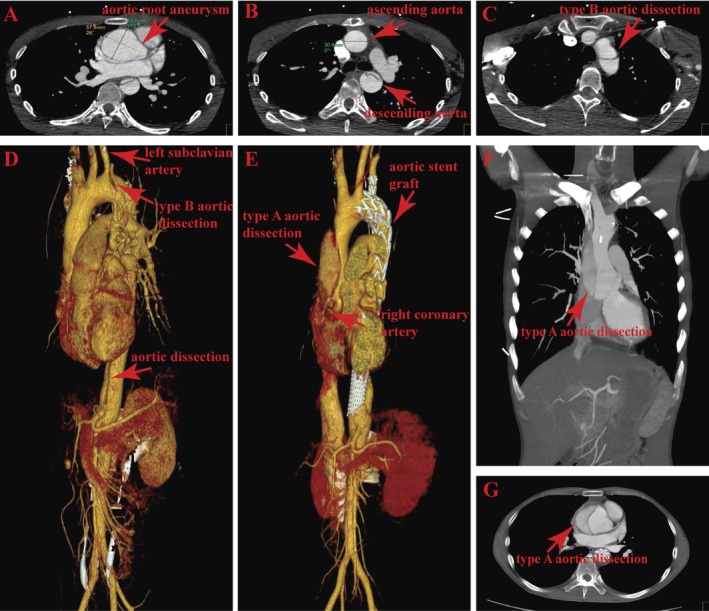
Computed tomography angiography images. (A, B) The maximum diameter of the aortic root aneurysm was 57.8 mm (arrow). (C, D) Imaging of type B aortic dissection (arrow). (E–G) Imaging of type A aortic dissection (arrow).
